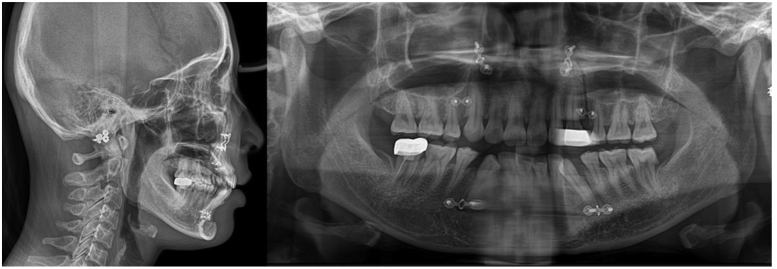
Pre-referral panoramic radiograph.
